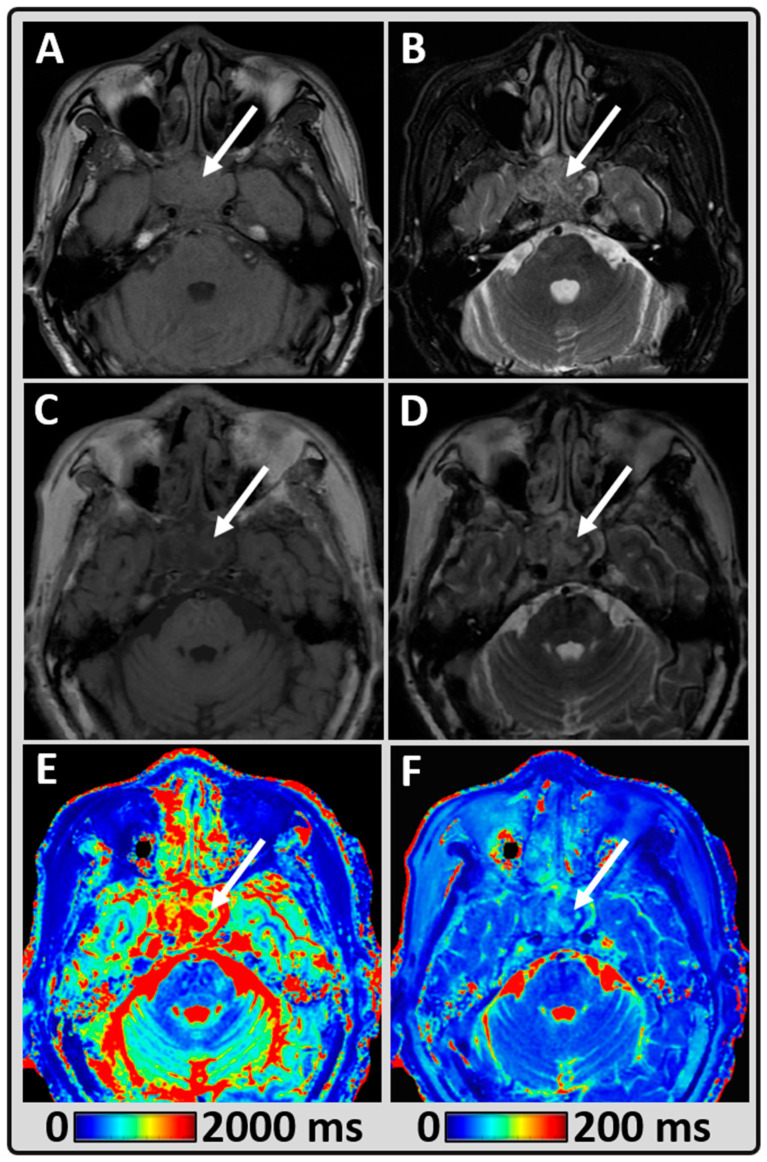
Representative MRI data from a 54-year-old male patient with white arrow pointing at recurrent nasopharyngeal cancer (post radiation therapy) extended to the sphenoid sinus. (A,B) Conventional qualitative pre-contrast T1w and T2w images depicting the lesion; (C,D) Synthetically generated T1w and T2w images using MAGiC; (E,F) MAGiC-generated quantitative T1 and T2 maps.
